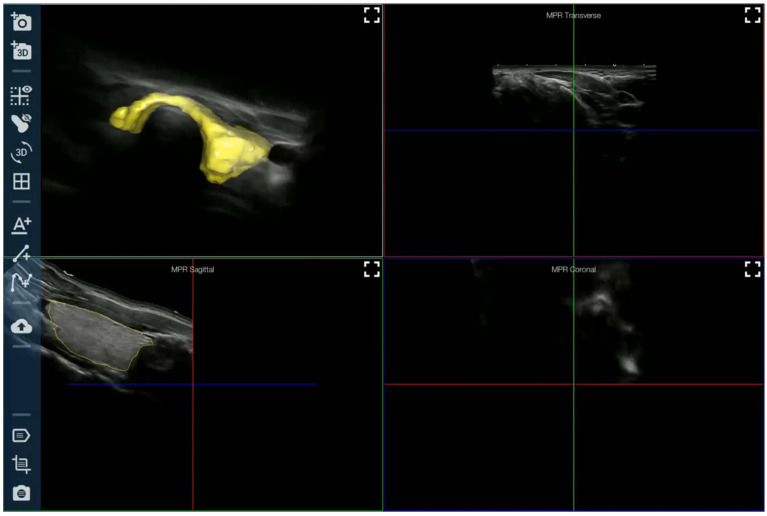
Three-dimensional representation of an ultrasound image of a thyroid nodule [32].
